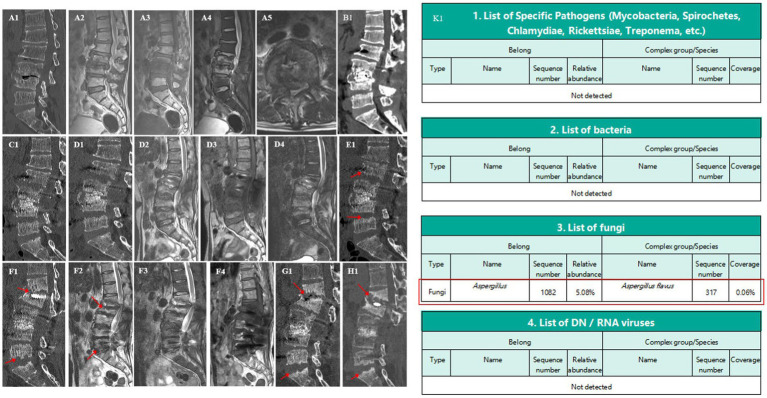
Spine CT/MRI and metagenomic sequencing. A1: moth-eaten changes at L3–L4, affecting discs and endplates. A2–A5: severe spinal canal occupation and potential spondylitis at L3–L4. B1,C1: progressive erosion in L4, L5, L2, and S1 endplates. D1–D4: relieved spinal canal occupation at L3–L4. E1: slow erosion and changes at the inferior endplate of L1. F1,G1: collapse of L1 anterior column and spinal canal mild stenosis. H1: stability and controlled inflammation. K1: Detection of *A. flavus*.
